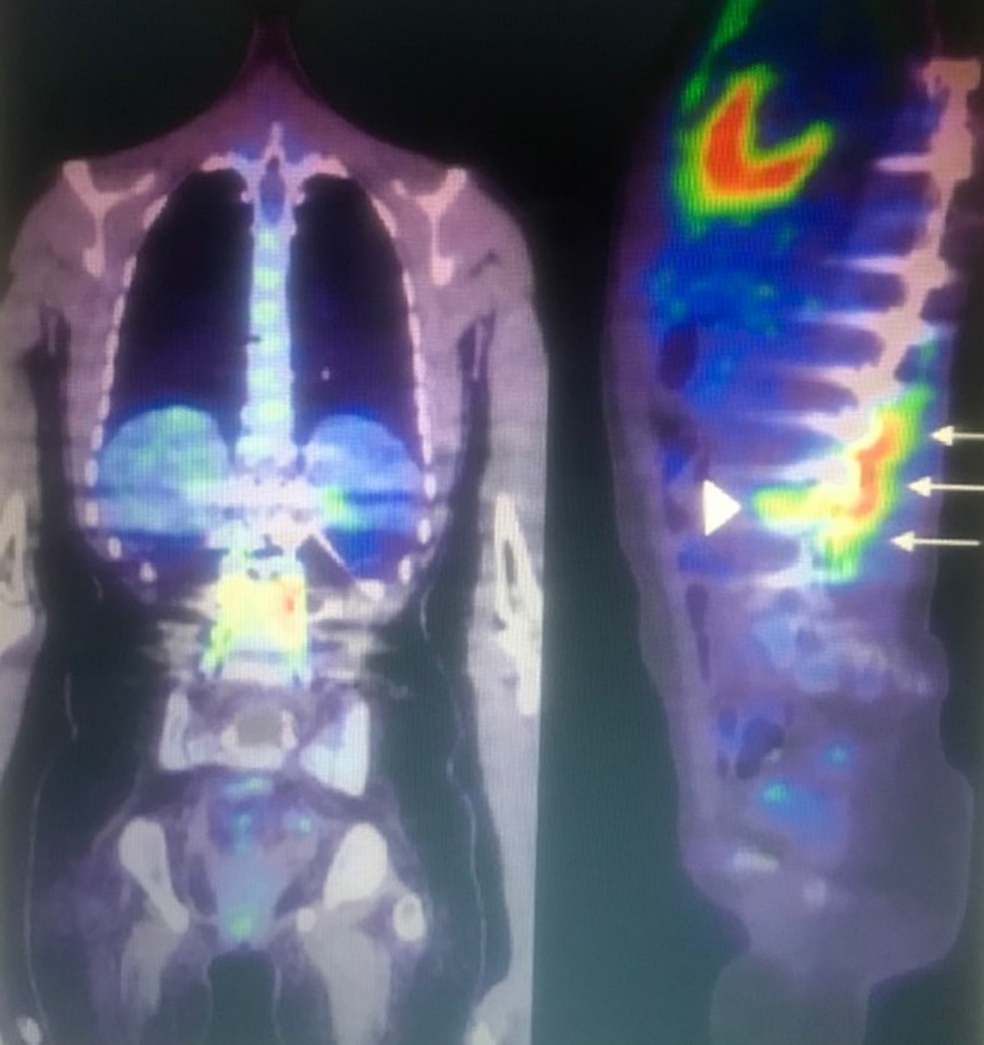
Thoracolumbar spinal hardware infection in a 14-year-old child. Coronal and sagittal 18f-FDG PET/CT views of the spinal hardware extending from T6 to L4. There is increased FDG uptake in the bone and soft tissues immediately adjacent to the hardware from T12 to L4 (arrows). FDG uptake at the bone hardware interface is present at the left L3 interpedicular screw (arrowheads). FDG, Fluorodeoxyglucose; PET, positron emission tomography; CT, computed tomography.
